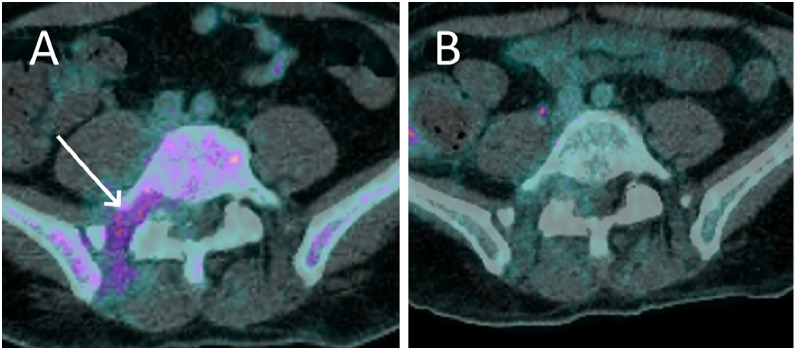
PET-CT image at S1 showing increased ^18^F-FDG uptake around the right paravertebral muscle and intervertebral foramen (A, arrow) corresponding to the location of the tumoural mass at one month after the initiation of treatment (A) and subsequent complete metabolic regression on the imagery performed two months after the initiation of treatment (B).
